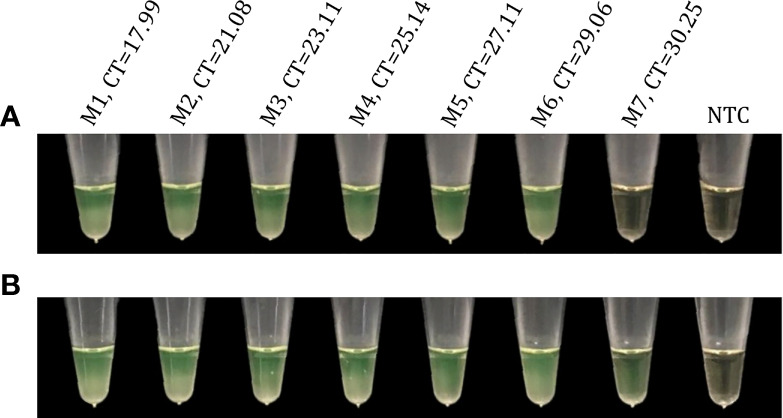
Evaluation of candidate primer sets for SARS-CoV-2 detection using a clinical sample panel with a viral load gradient. Reactions using the candidate primer sets R18-8N3-R34 (A) and R16.2-R34-R2 (B) were considered positive for RT-LAMP products if they had a color change from brown to green. Viral RNA obtained from clinical samples were analyzed in parallel by multiplex RT-LAMP and RT-qPCR assays. M1-M7, clinical samples with different cycle threshold (CT) values obtained by RT-qPCR; NTC, negative non-template control.
